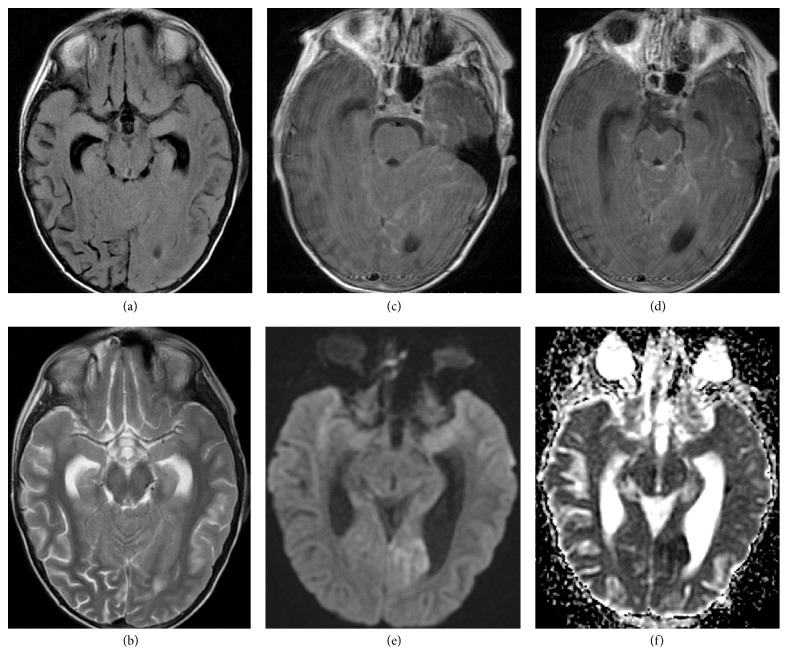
A 7 year-old boy with wrist rhabdomyosarcoma. The metastatic spread is seen only in the leptomeninges. MRI; FLAIR (a); FSE/T2WI (b); SE/T1WI after gadolinium administration (c, d); DWI (e); ADC map (f).
